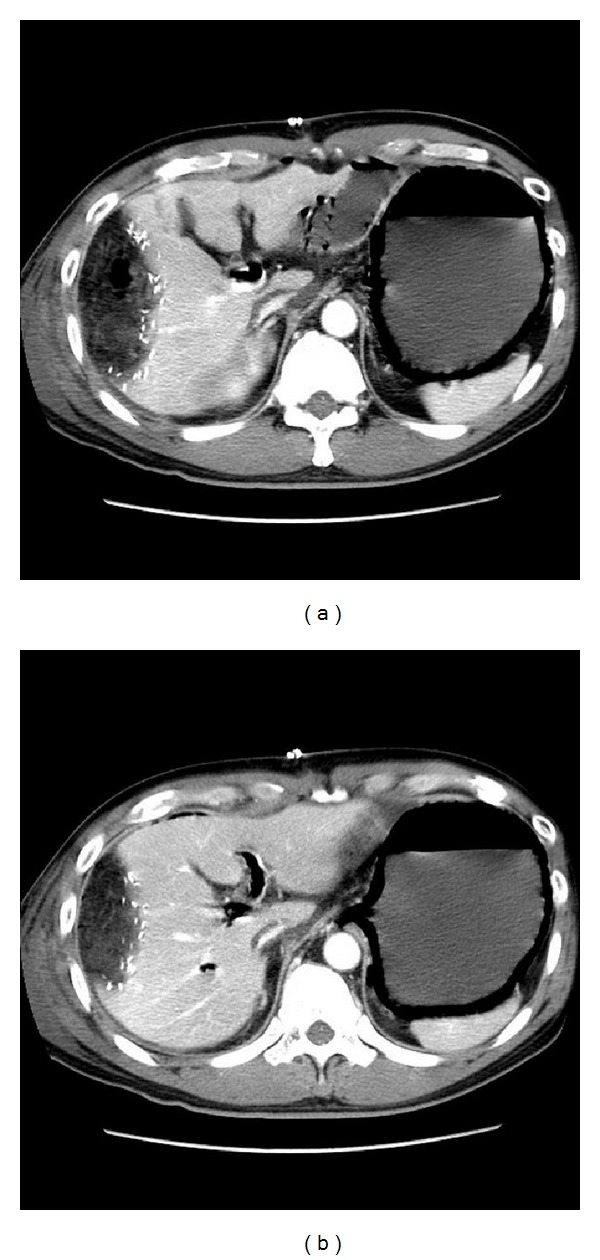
CT image confirming presence of gas in stomach wall. Gas is also noted in the portal vein.
